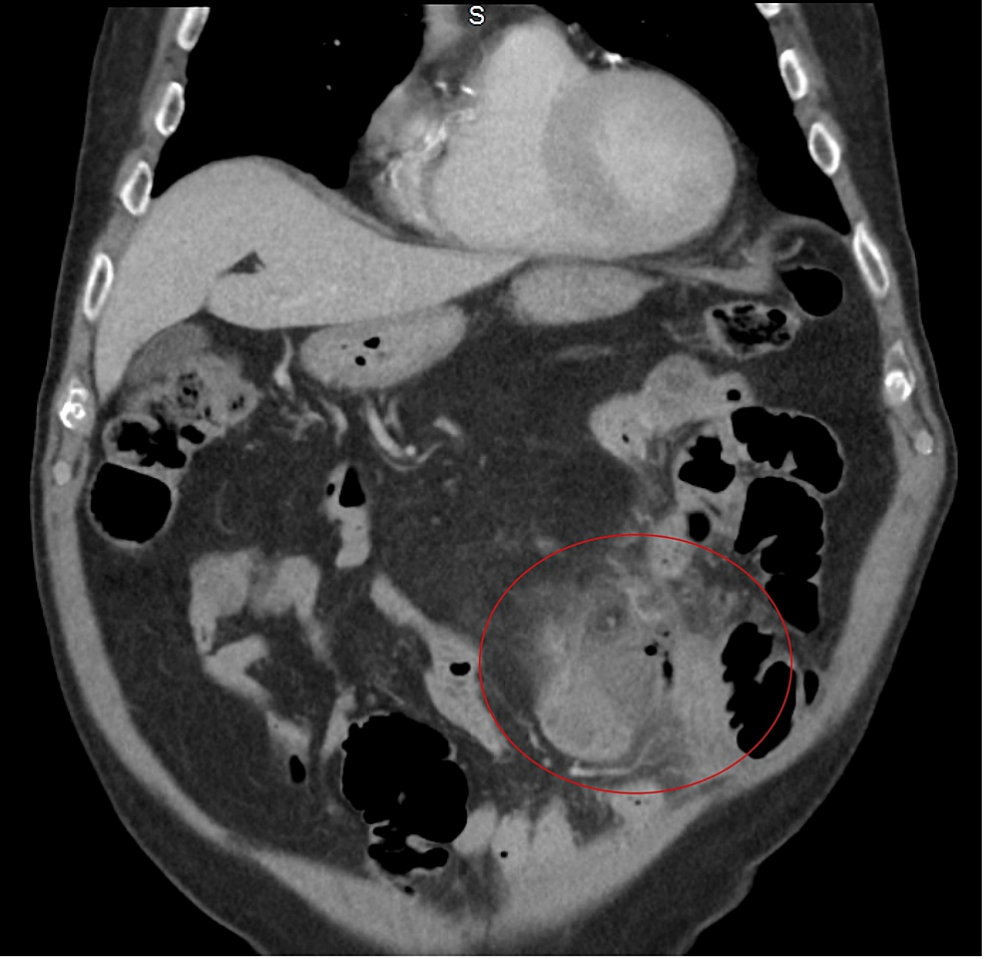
A coronal CT image showing bowel perforation on the left side of the abdomen (red circle). This appears to involve the small bowel, with surrounding inflammatory reaction and extraluminal air, but there is no evidence of a definable abscess, and no distant free intraperitoneal air is visible.
